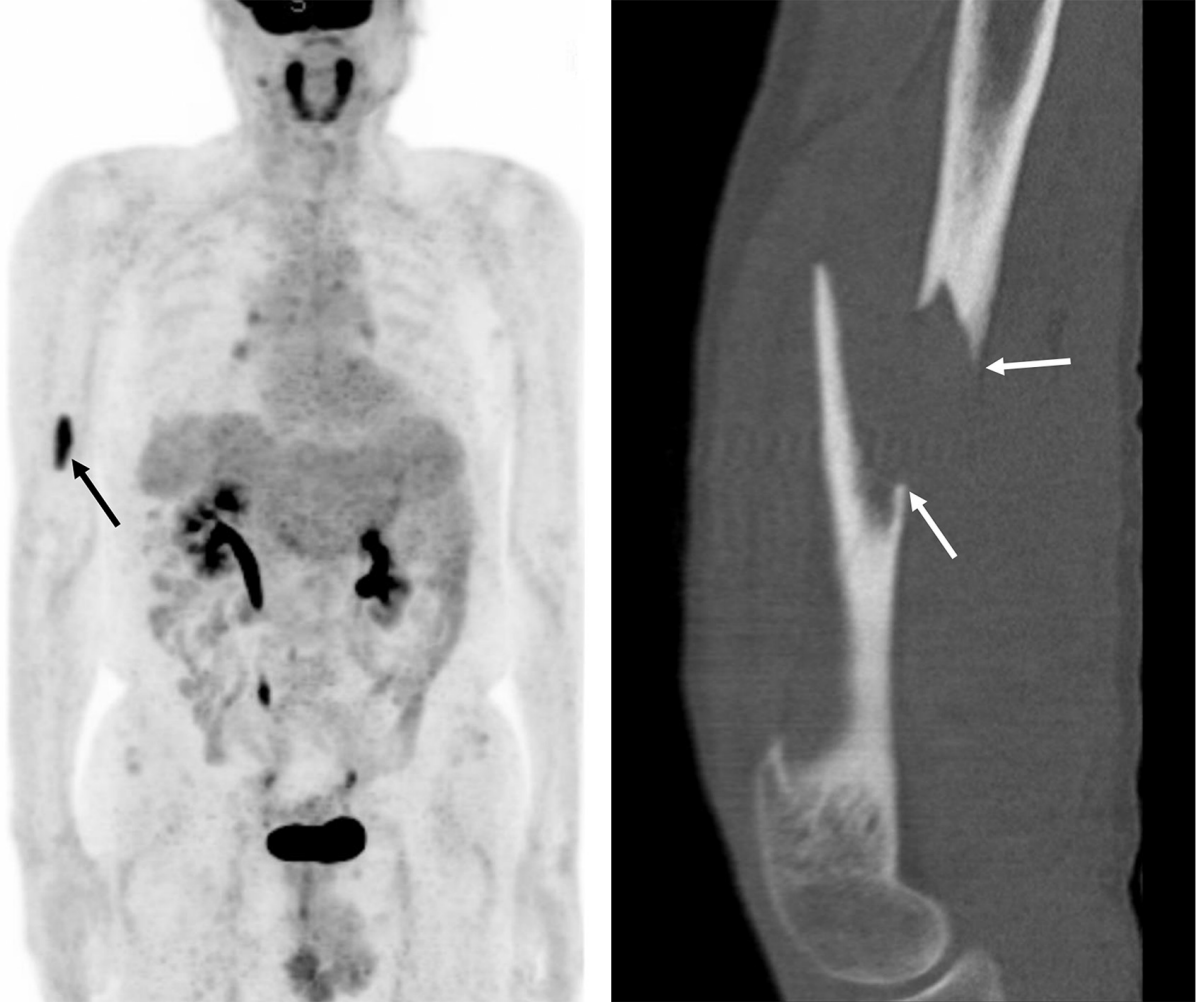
A 76-year-old man who had undergone radiofrequency ablation, transarterial chemoembolization, and proton beam radiation therapy. After 7 months, the tumor marker levels were remeasured (AFP: 2.6 ng/ml; PIVKA-II: 123 mAU/ml), while MRI did not reveal a recurrent lesion. A thorough evaluation was performed including 2 liver MRI scans within 105 days and CT of the abdomen and chest, but all were negative. (a) PET-CT (3-dimensional maximum intensity projection imaging) performed 107 days after elevated tumor marker levels were first observed, showing metastasis (arrow) at the right humerus. (b) CT scan of the right humerus obtained 5 days after PET-CT when a non-traumatic pathologic fracture (white arrow) occurred during regular physical activity.
